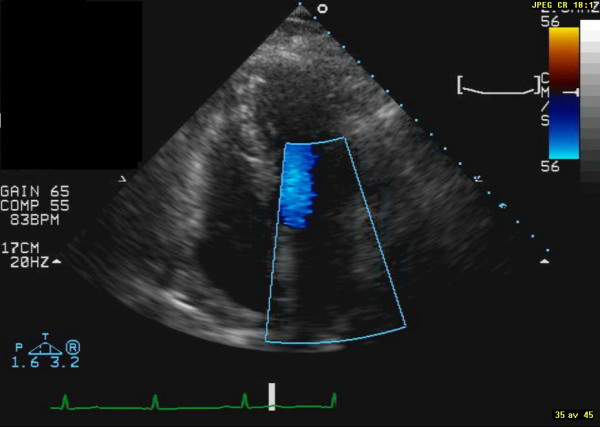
Display of end-systolic image from apical 4-chamber view before treatment with pioglitazone.
